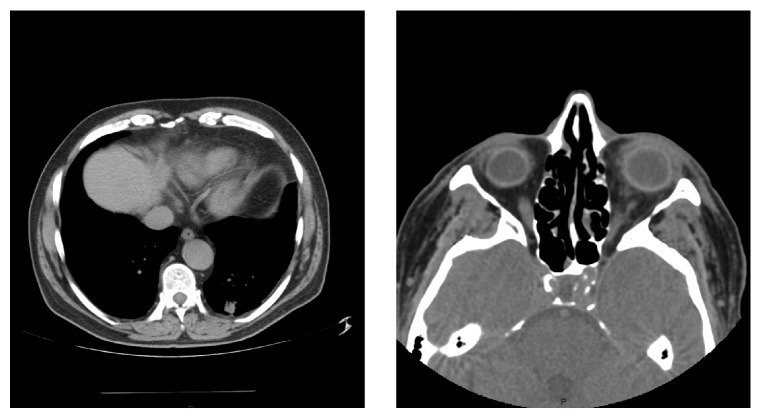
Orbital and lung CT scan.
